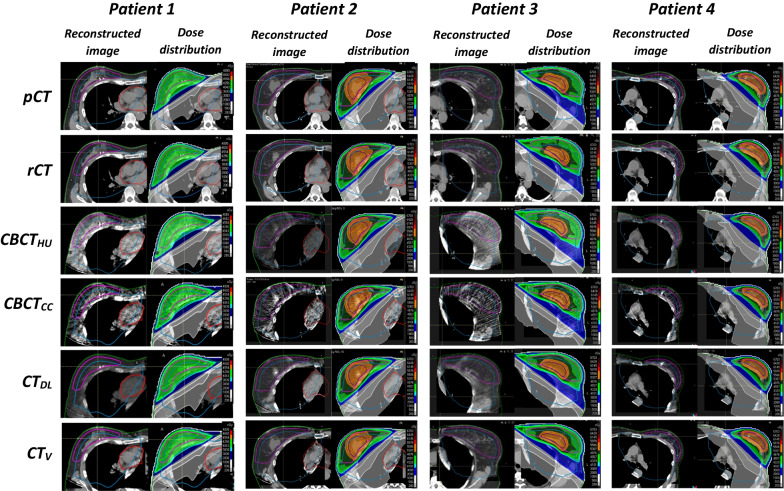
Overview of the different images used during analysis for four different patients. The images show the different ROIs used during analyses, including the calculated dose distribution according to the original treatment plan. The W/L was set to 400/40HU. The ROIs present are External (green), whole breast CTV (pink) boost CTV (pink), heart (red) and CBCT-ROI (blue).The ROIs are warped from the rCT, hence the differences between the image sets
